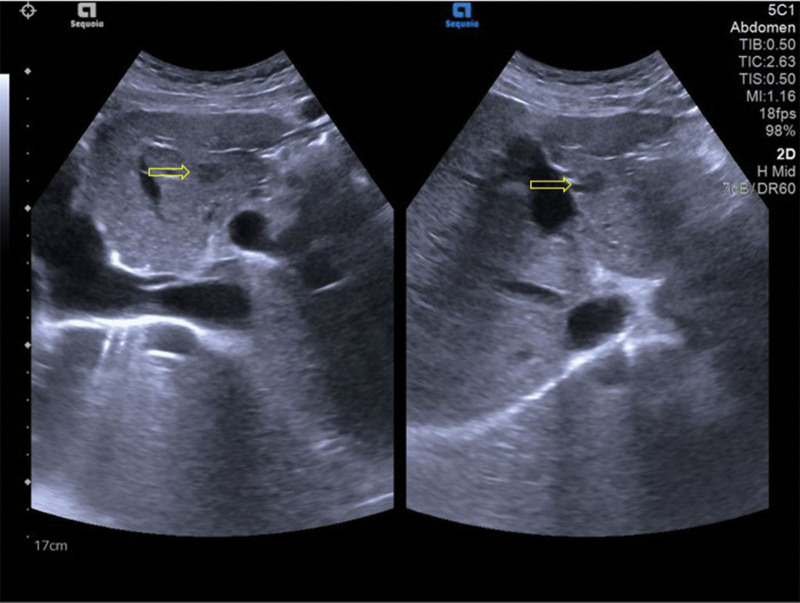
The results of two-dimensional ultrasound examination of the liver. The shape and section of the liver were normal, the edge of the liver was smooth, the light spots in the liver were thickened and enhanced, the distribution was uneven, and the blood vessels are clear. Multiple hypoechoic nodules can be seen in the liver (indicated by the yellow arrow), with clear contours and uneven internal echoes.
